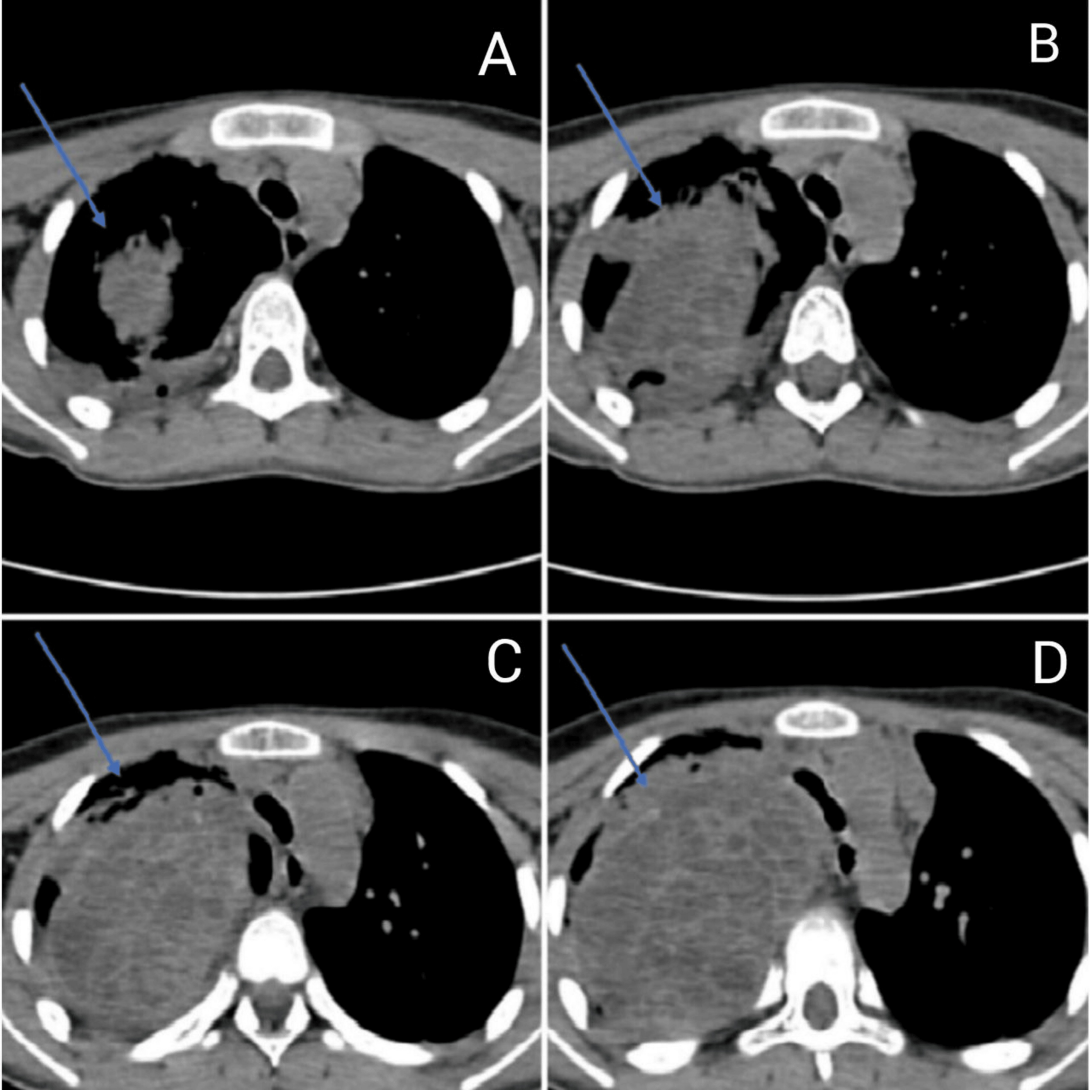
HRCT scan showing mass (blue arrows) at various levels: A) upper part of trachea; B) lower part of trachea; C) just above the carina; D) at the level of carina HRCT, high-resolution computed tomography.
